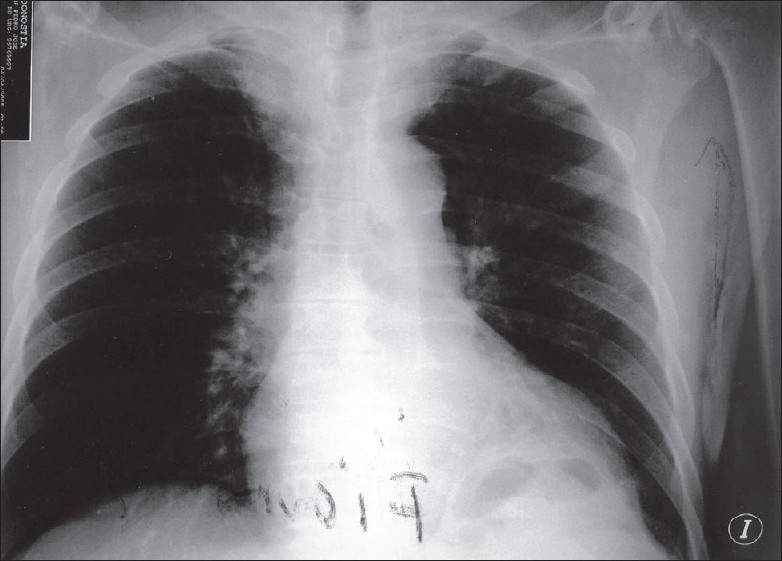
The chest X-ray on admission. PA view
